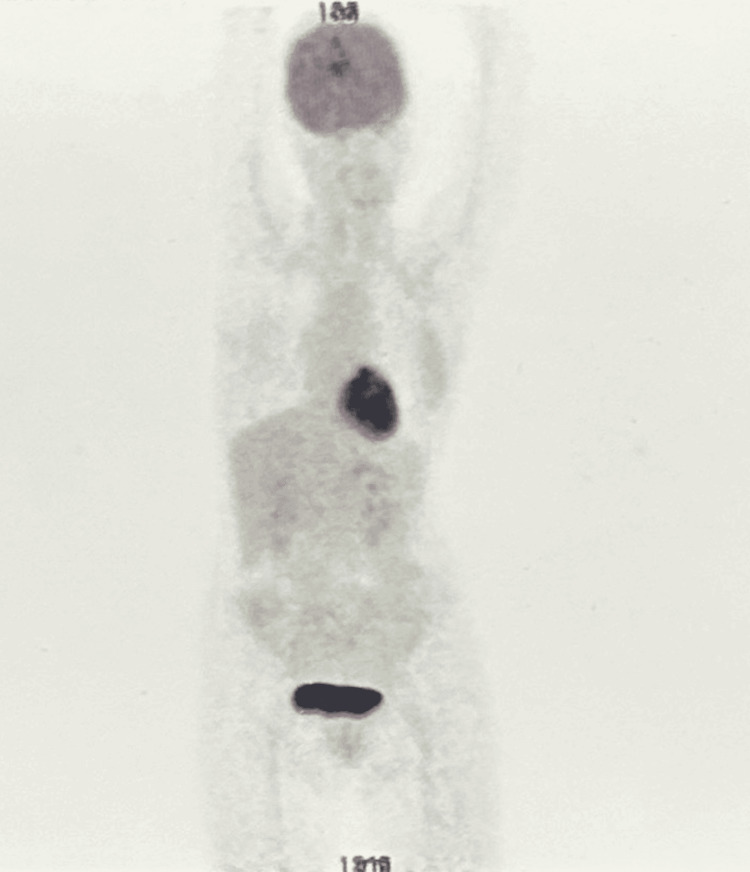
Whole-body PET scan showing no obvious FDG-avid lesions, thereby ruling out metastasis PET: positron emission tomography, FDG: fluorodeoxyglucose
